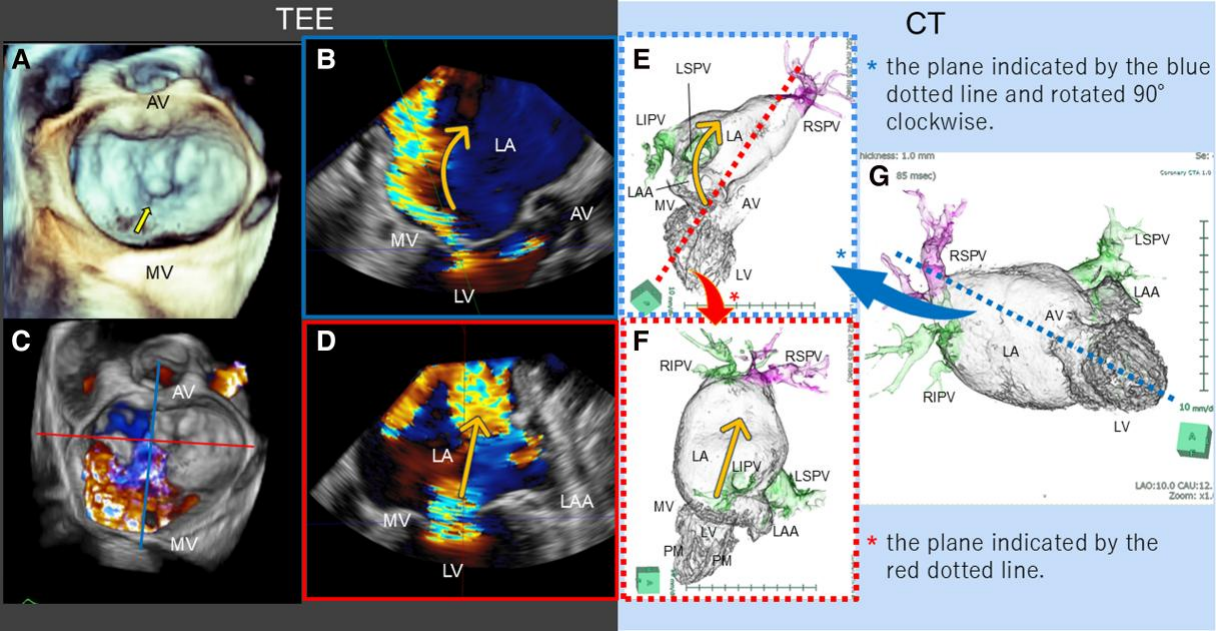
Anatomical relationship between the mitral regurgitation jet and the pulmonary veins on 3D transoesophageal echocardiography and contrast-enhanced computed tomography. (*A, C*) Three-dimensional transoesophageal echocardiography (3D transoesophageal echocardiography) with en face views of the mitral valve, showing A2 prolapse with chordal rupture (yellow arrow) (*A*) and eccentric severe mitral regurgitation (*C*). (*B, D*) 3D transoesophageal echocardiography colour Doppler of the mitral regurgitation jet due to A2 prolapse, displayed in the long-axis view (*B*) and commissure view (*D*). The long-axis view view (*B*) is obtained by sectioning along the blue line indicated on (*C*) (multiplanar reconstruction), and the commissure view (*D*) is obtained by sectioning along the red line on (*C*). Orange arrows indicate mitral regurgitation jet direction. (*G*) Volume-rendered reconstruction from contrast-enhanced computed tomography in left anterior oblique 10° and caudal 12° projections, depicting all four pulmonary veins, left-sided cardiac structures, and the aorta. The right superior pulmonary vein was depicted in purple, whereas the remaining pulmonary veins were depicted in green. (*E*) Multiplanar reconstruction along the blue dotted line in (*G*), rotated 90°clockwise. Orange arrows indicate mitral regurgitation jet direction. (*F*) Multiplanar reconstruction along the red dotted line in (*E*). Orange arrows indicate mitral regurgitation jet direction. *Panel correspondence and key finding*. Matched cross-sectional planes from 3D transoesophageal echocardiography colour Doppler and computed tomography multiplanar reconstruction are displayed side-by-side to demonstrate the jet direction. (*B*) corresponds to (*E*), and (*D*) corresponds to (*F*). Across these matched planes, the eccentric mitral regurgitation jet was directed towards the right superior pulmonary vein. AV, aortic valve; LA, left atrium; LV, left ventricle; LAA, left atrial appendage; LIPV, left inferior pulmonary vein; LSPV, left superior pulmonary vein; MV, mitral valve; RIPV, right inferior pulmonary vein; RSPV, right superior pulmonary vein; PM, papillary muscle.
